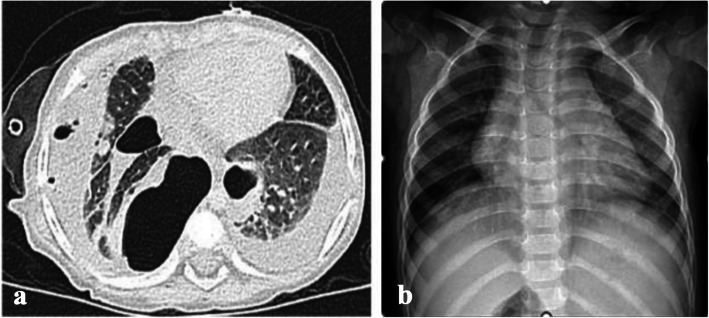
18 months old infant presented with history of high-grade fever, oral thrush and dyspnea for 2 weeks. She presented to the A&E with cyanosis and marked respiratory distress. She had tension pneumothorax on the right side requiring urgent decompression, followed by chest drain insertion. Pleural fluid culture showed growth of MRSA and sputum culture showed growth of Klebsiella pneumoniae. a CT chest showed bilateral necrotizing pneumonia. Immunodeficiency, HIV and TB were excluded. She showed good clinical recovery after 3 weeks of IV antibiotics, with regression of inflammatory markers and radiological improvement. b The pulmonary lesions resolved completely at the 1 year follow up
